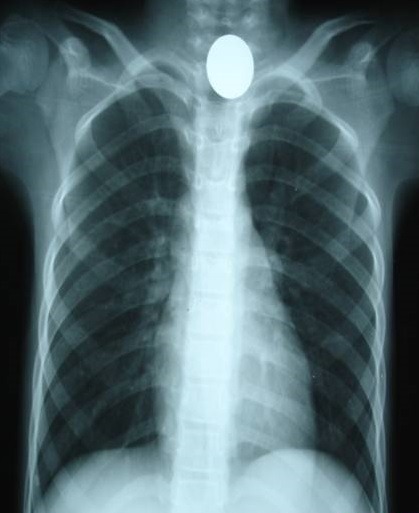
Radiographie thoracique objectivant un corps étranger radio-opaque œsophagien (pièce de monnaie)
